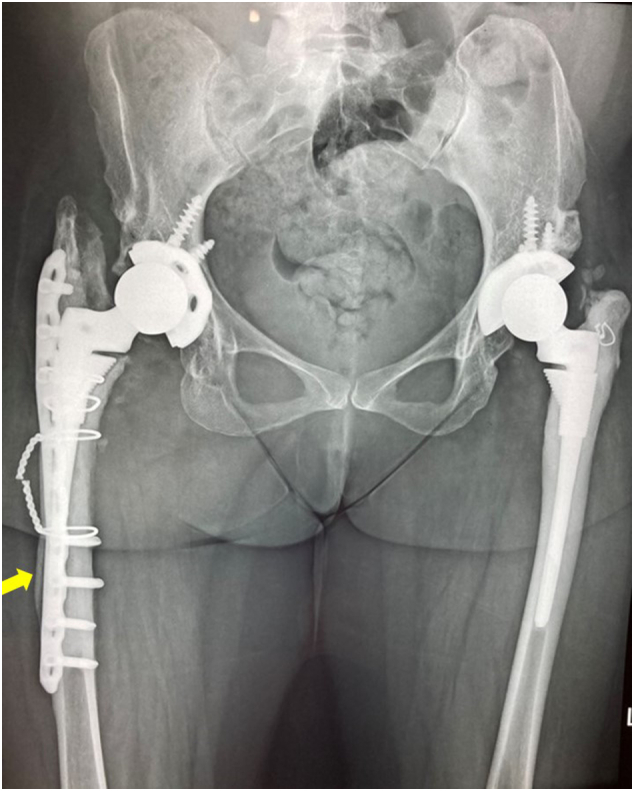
X-rays taken 5-years after the second arthroplasty. Note the healed fracture indicated by the arrow.
